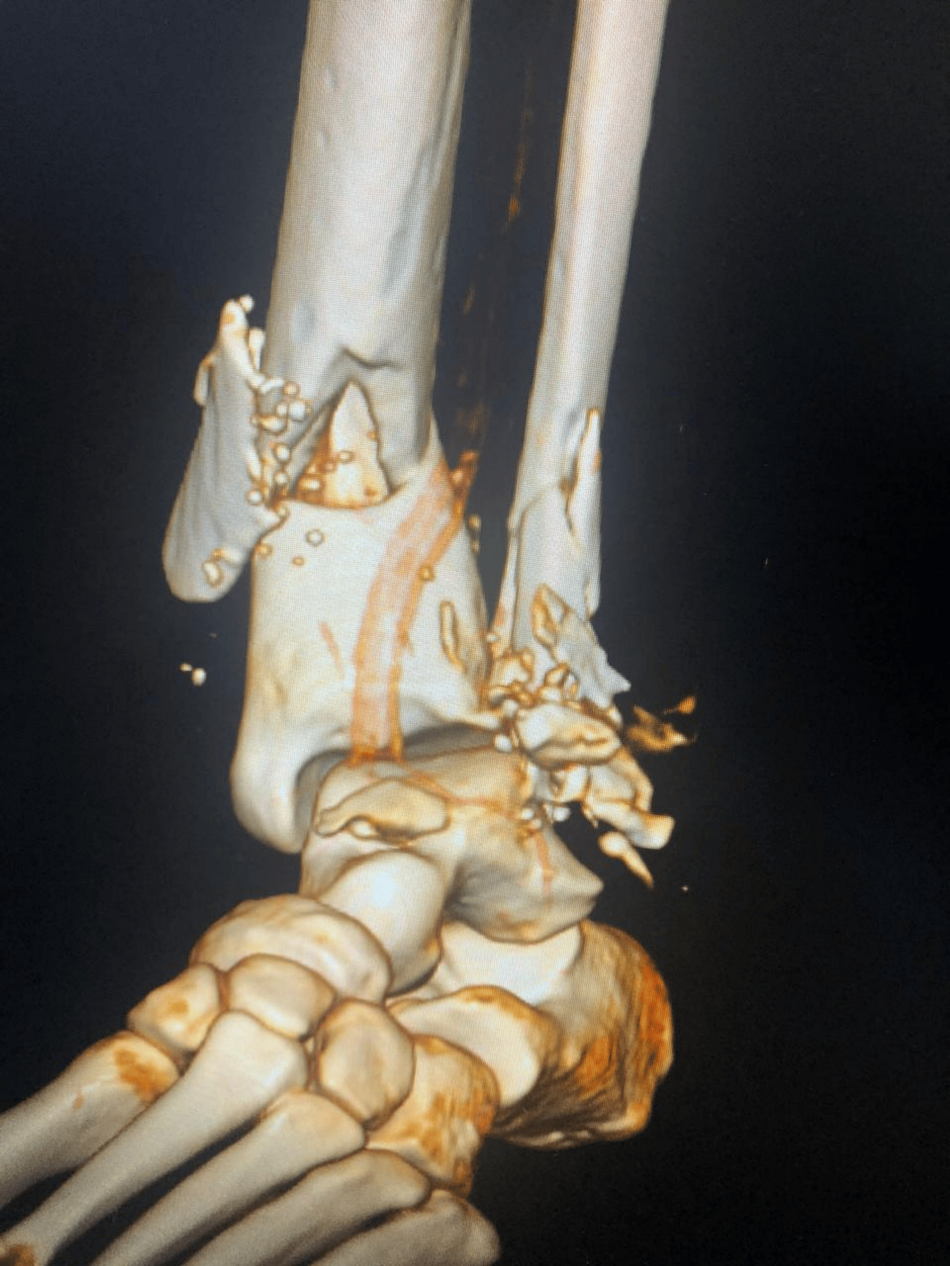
Three-dimensional CT scan view showing comminuted distal fibula fracture and distal shaft tibia fracture
